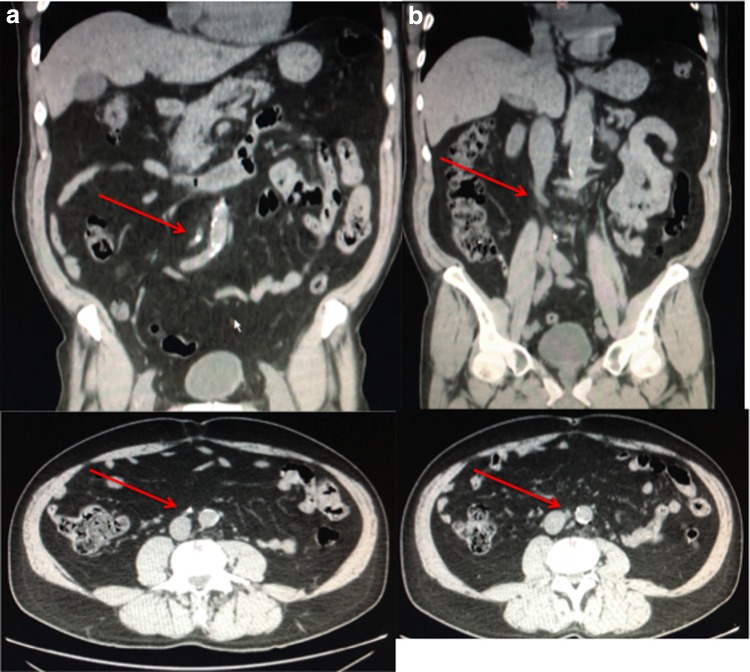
(a) Preoperative CT scan (*red arrow* shows the stone); (b) preoperative CT scan (*red arrow* shows the retrocaval ureter).
